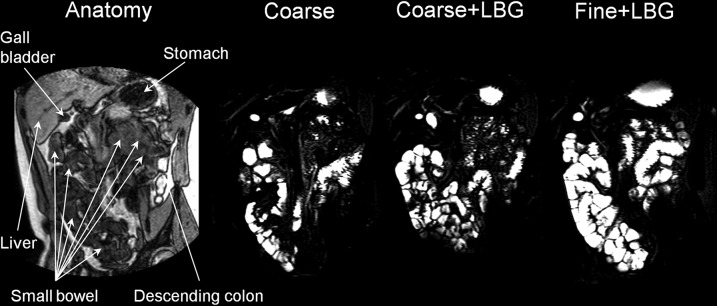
On the left side of the panel is a coronal anatomic “roadmap” dual-echo MRI image. The other images are corresponding small bowel water images taken across the small bowel of the same healthy young adult at time t = 4 h after consumption of each of the 3 meals containing a 20% sunflower oil in water emulsion on the 3 separate study days: Coarse, Coarse+LBG, and Fine+LBG. These images show the large amount of freely mobile fluid present in the small bowel in response to the fat emulsion meals. Coarse, 20% oil and water emulsion with 6-μm mean droplet size; Coarse+LBG, 20% oil and water emulsion with 6-μm mean droplet size and 0.5% locust bean gum; Fine+LBG, 20% oil and water emulsion with 0.4-μm mean droplet size and 0.5% locust bean gum; LBG, locust bean gum.
